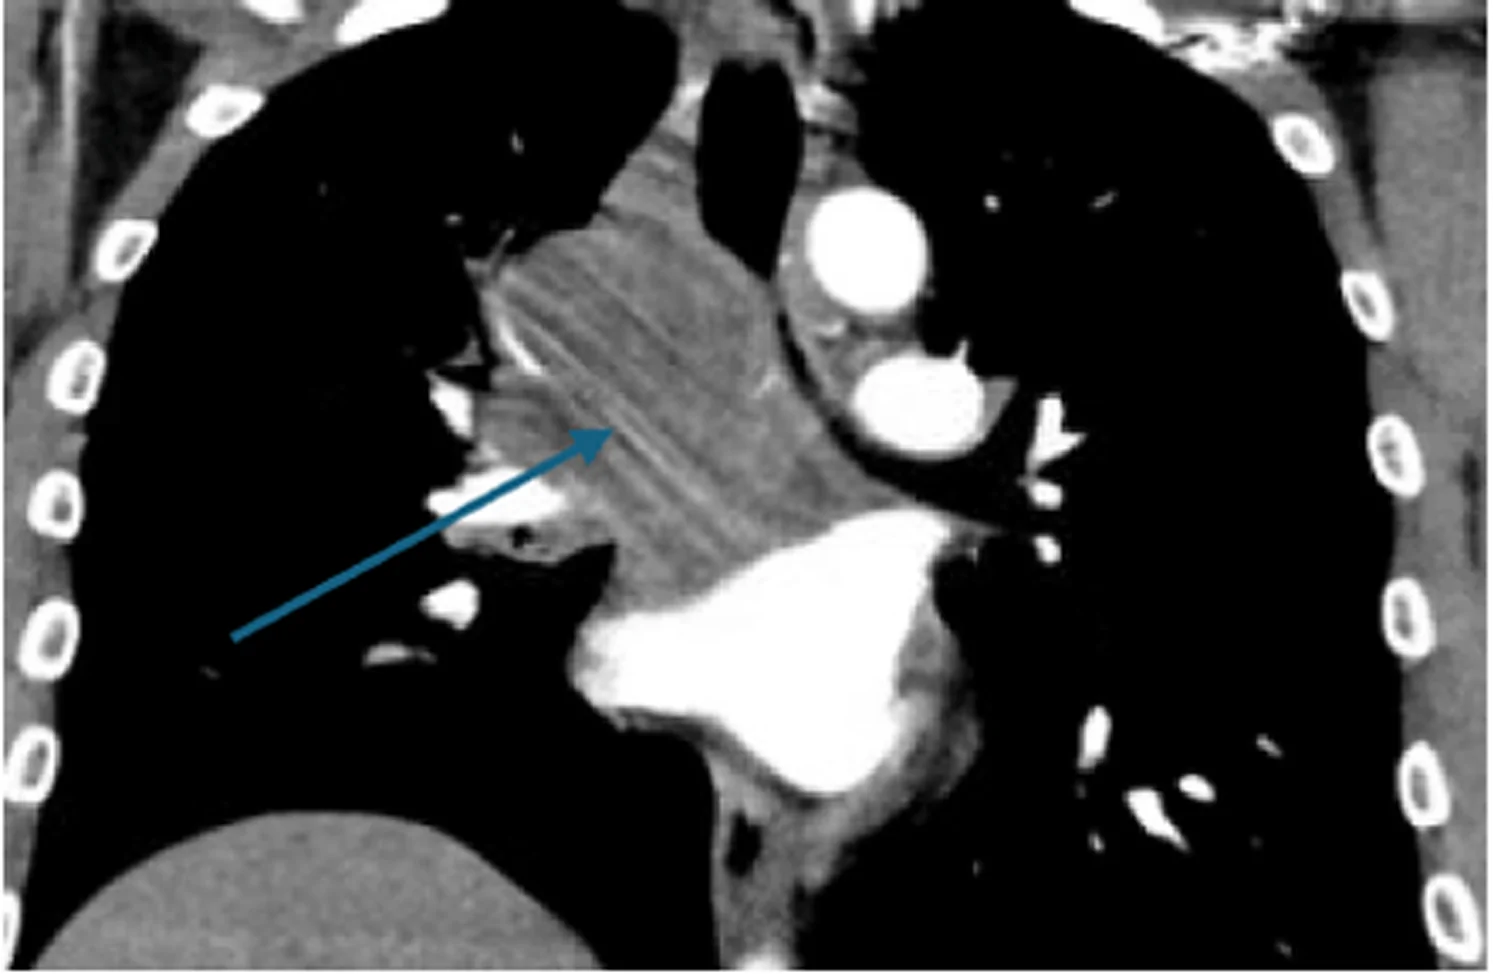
Coronal section of the CT angiography chest showing mediastinal adenopathy (blue arrow) with encasement of right-sided pulmonary arteries and right middle and lower lobe bronchi with obstruction of the right middle lobe bronchus.
